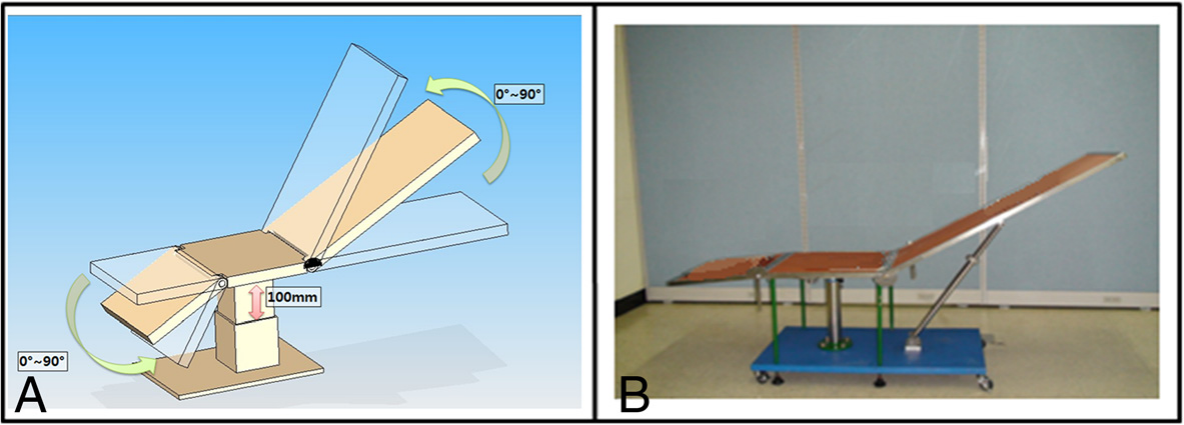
A simplified chair device with three-segmental rigid plates for evaluating sagittal alignment with radiography. (A) The chair was designed with a leg rest, a seat, and a seat back comprising three separate parts. The length, width, and thickness of the seat back, seat, and leg rest were 970 × 400 × 20, 500 × 400 × 20, and 450 × 400 × 20 mm, respectively. (B) Prototype of the chair device.
